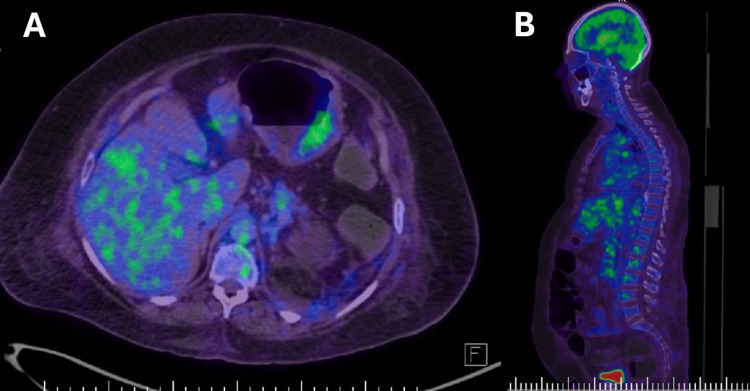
18F-FDG PET-CT images (A) Axial (transverse) PET-CT slice of the upper abdomen; (B) Sagittal PET-CT slice of the whole body. 18F-FDG: fluorine-18 fluorodeoxyglucose
